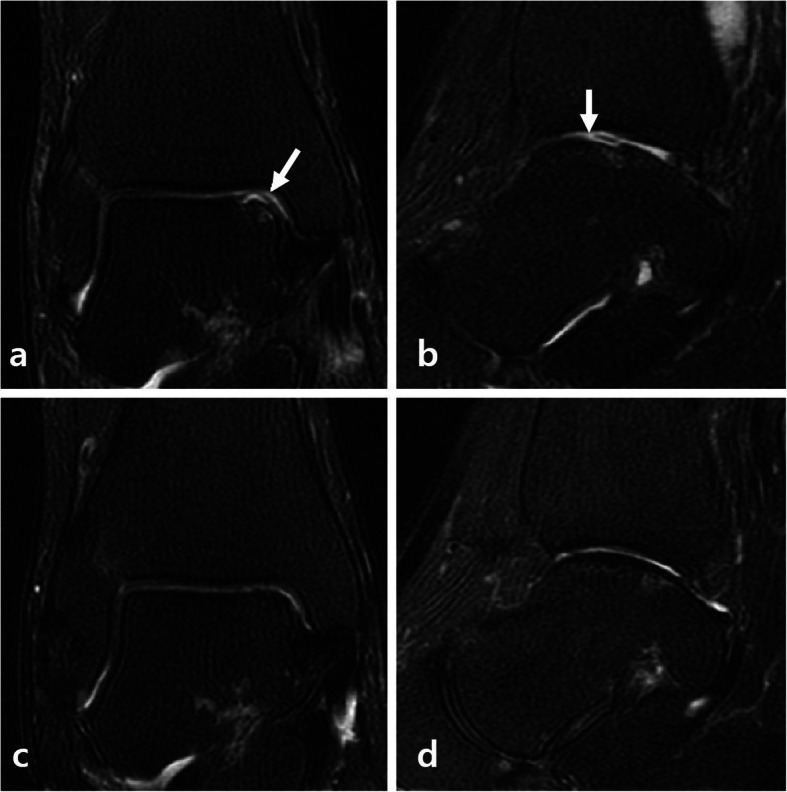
Preoperative magnetic resonance imaging (MRI) showing (a-b) an osteochondral lesion of the talus (arrow). c-d, Complete filling of the osteochondral defect 2 years after arthroscopic microfracture with atelocollagen augmentation
